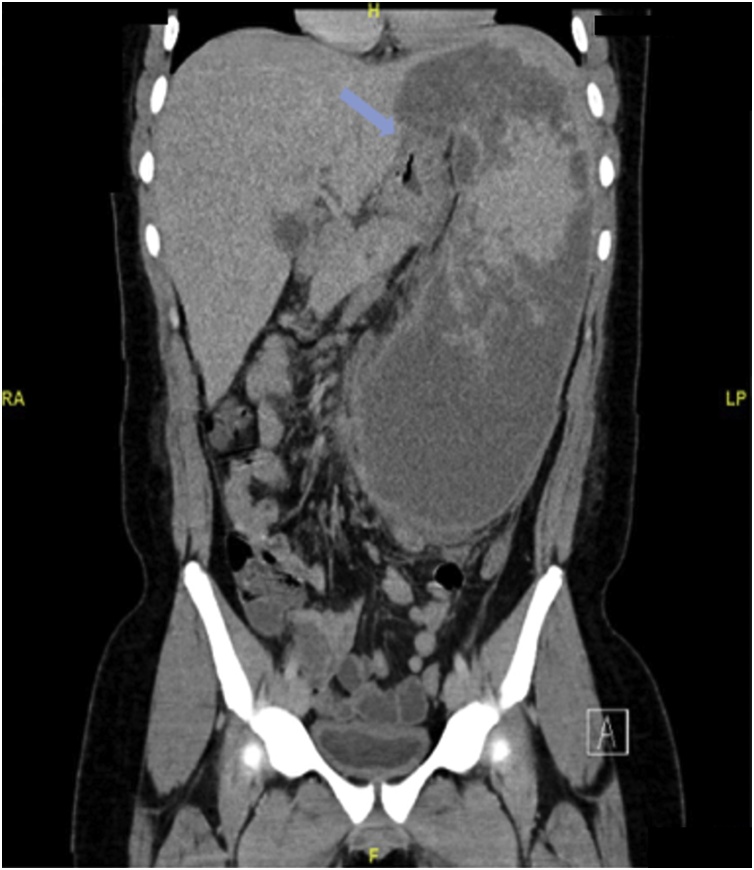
Coronal view of abdominal CT scan with IV contrast showing massive splenomegaly with query indentation of upper part of the stomach.
